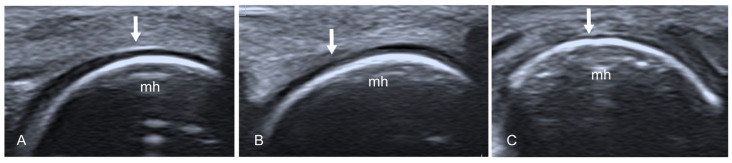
Reference ultrasound images of metacarpophalangeal joint cartilage damage according to the OMERACT scoring system. (A) Grade 0: Normal appearance. (B) Grade 1: Minimal changes. (C) Grade 2: Severe changes. OMERACT, Outcome Measures in Rheumatology; arrows, margin between cartilage and synovium; mh, metacarpal head.
